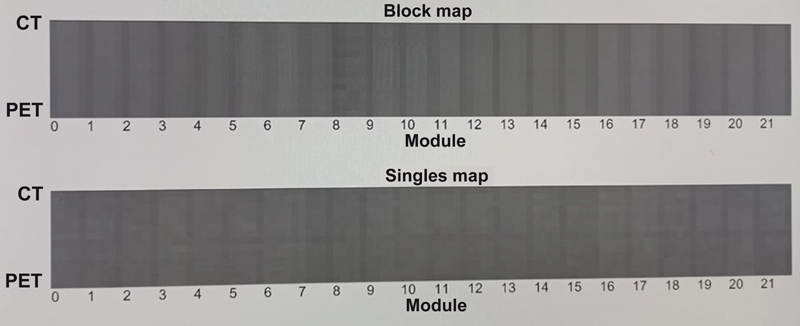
Block image. CT, computed tomography; PET, positron emission tomography.
